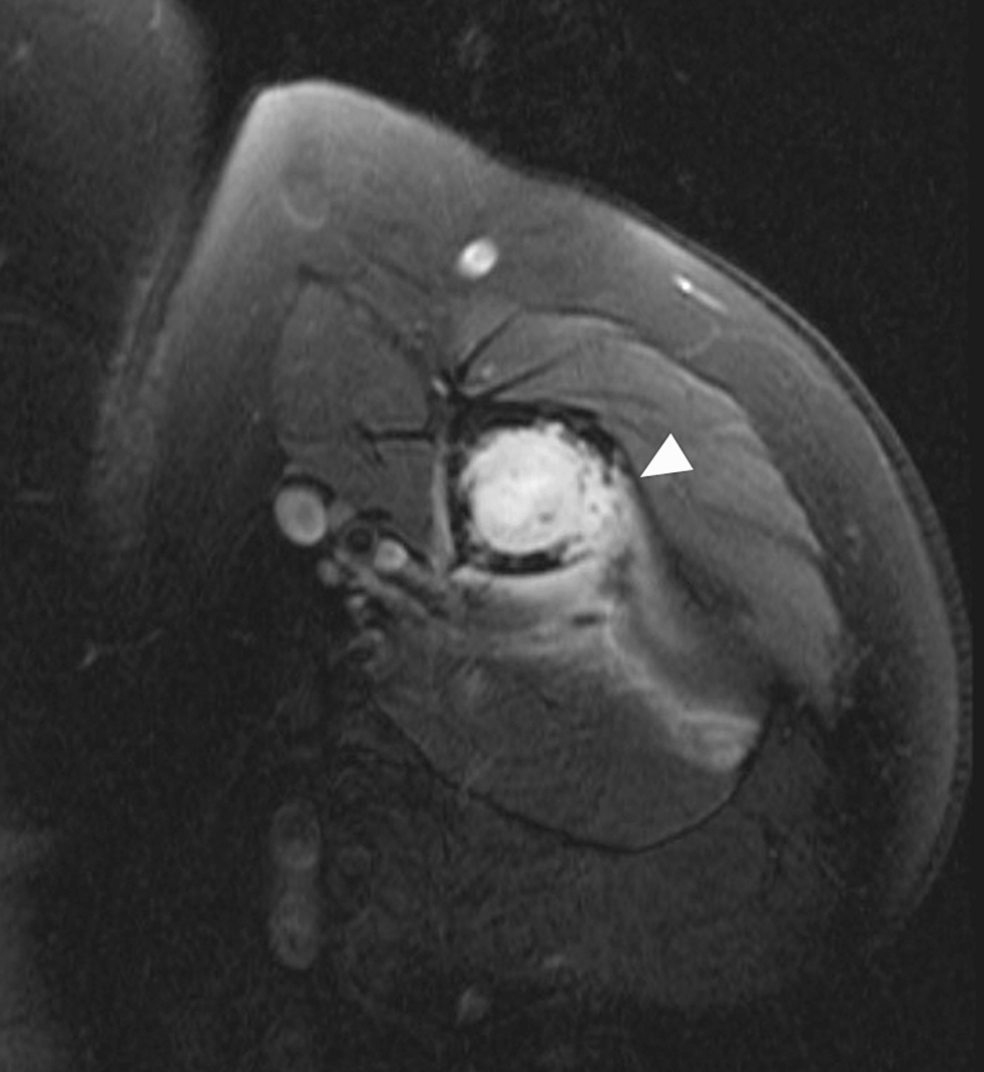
T2-weighted, fat-suppressed MRI image of the left humerus with white arrow demonstrating osseous metastases. MRI, magnetic resonance imaging.
